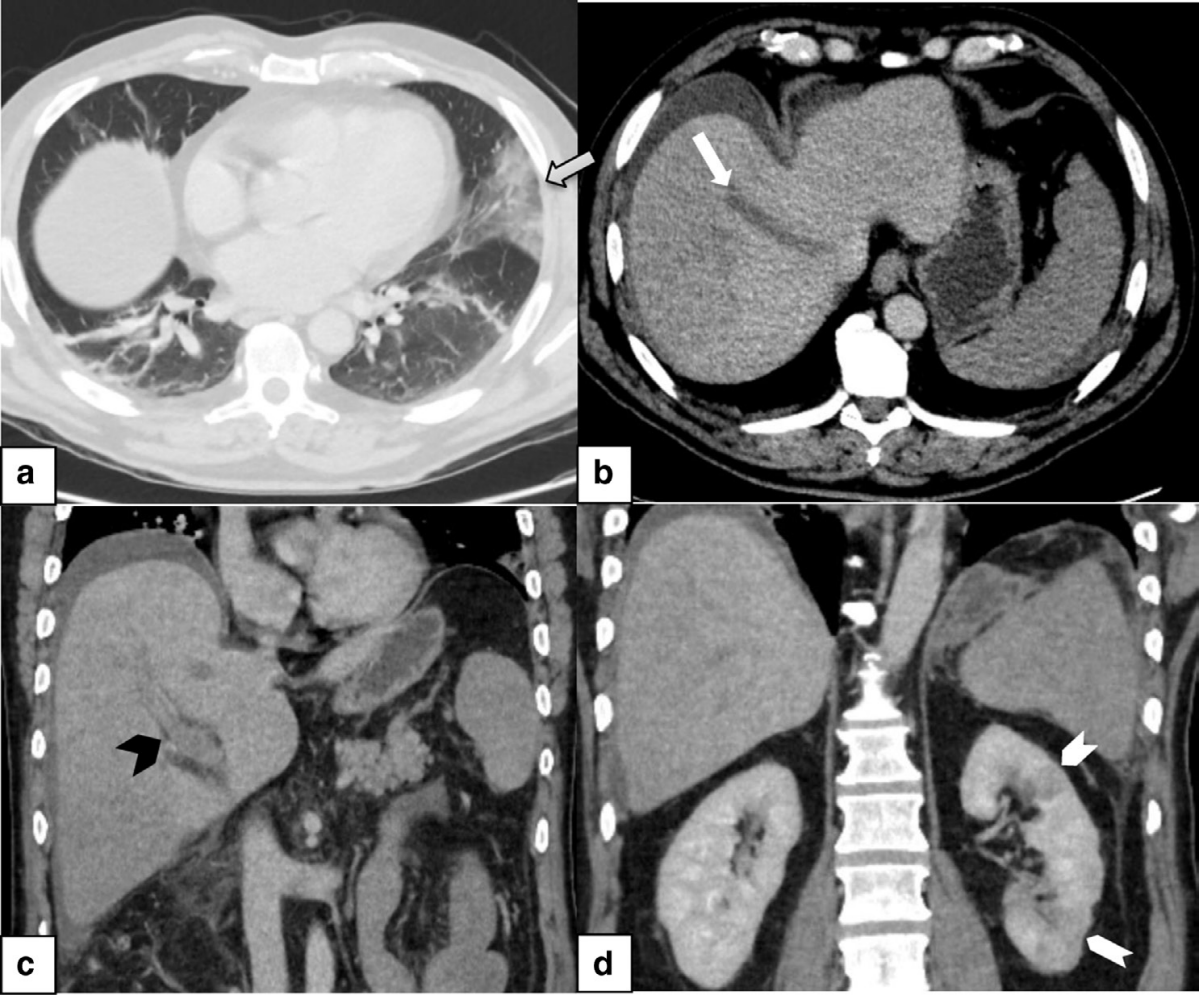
CT in portovenous phase shows (a) a wedge-shaped infarct (grey arrow) in the linguar segment of the lung (b) axial section of the liver with a thrombus in the middle hepatic vein (white arrow). Coronal images of the abdomen demonstrate (c) portal vein thrombosis (black arrowhead) and (d) infarcts in the left kidney (white arrowheads).
